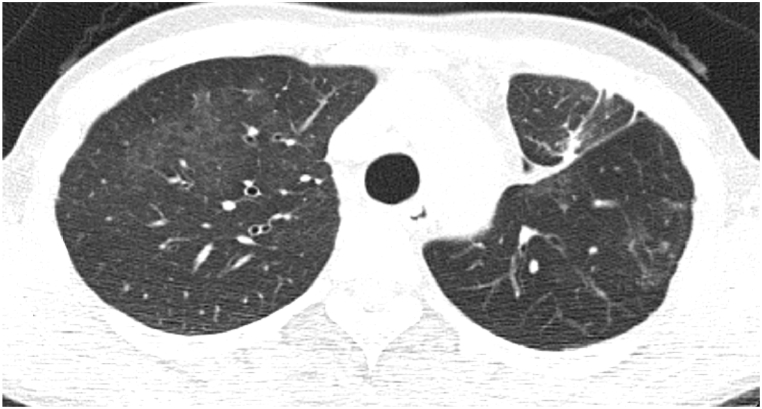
Chest CT during the second hospitalization for pneumonia.
